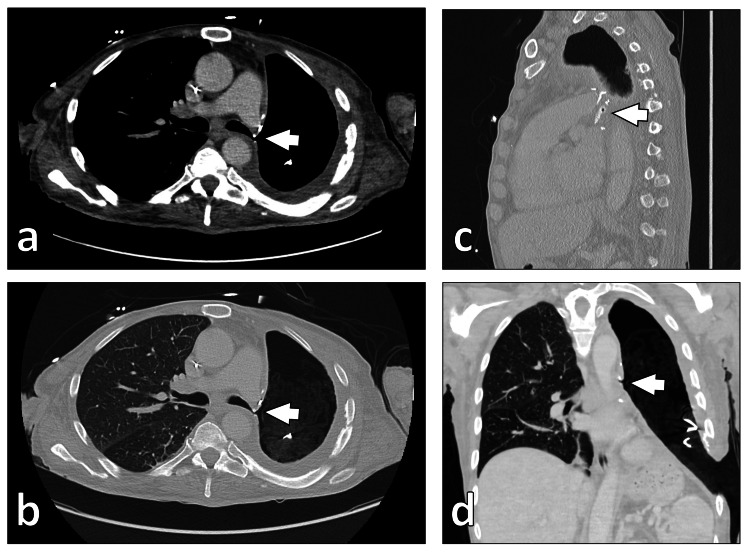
CT scan demonstrating bronchial stump insufficiency (indicated by the arrow) following pneumonectomy. (a, b) Transversal views. (c) Sagittal reconstruction. (d) Coronary reconstruction. CT, computed tomography
